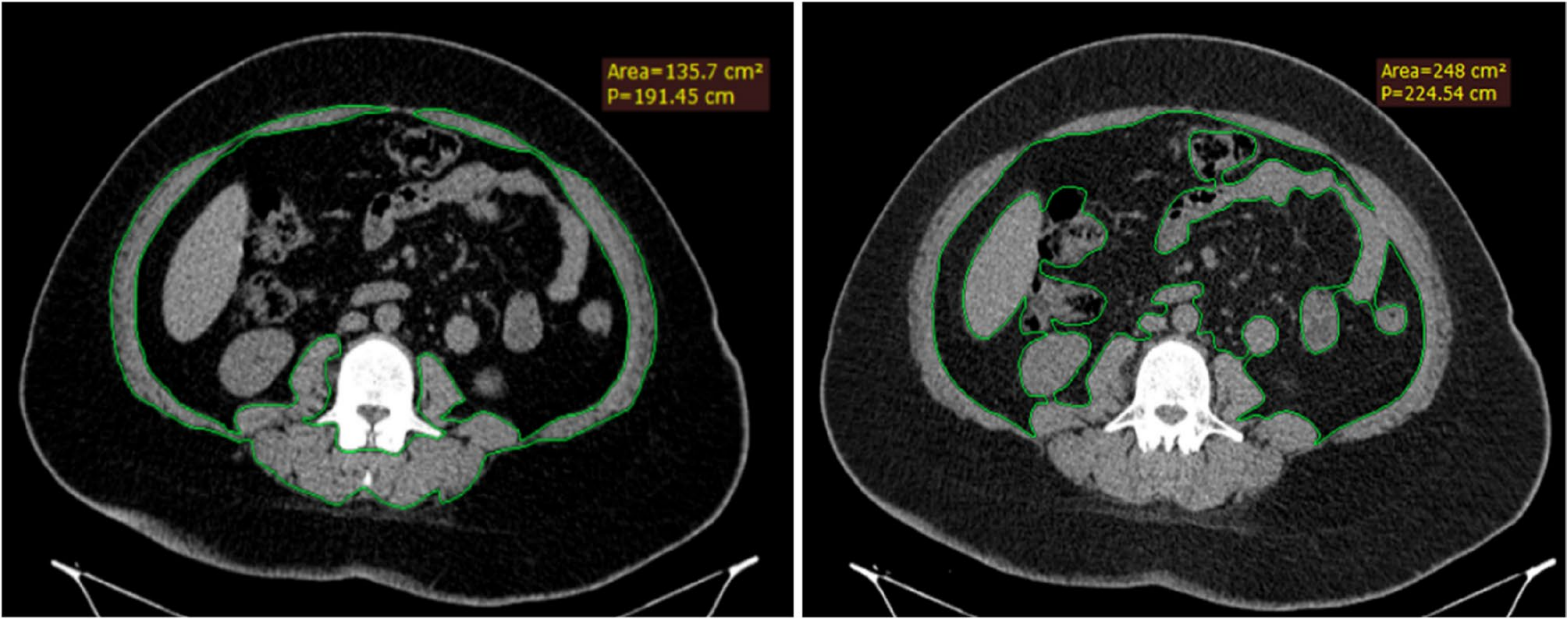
Preoperative non-contrast CT scan from the control arm; TAMA and VFA calculated by manually tracing the total visible abdominal muscles at L3 vertebra as shown. a TAMA = 135.7 cm^2^ b VFA = 248 cm^2^. Given the patient’s height of 1.58 m, TAMAI was estimated 54.4 cm^2^/m^2^ and VFA/TAMAI ratio 4.6
